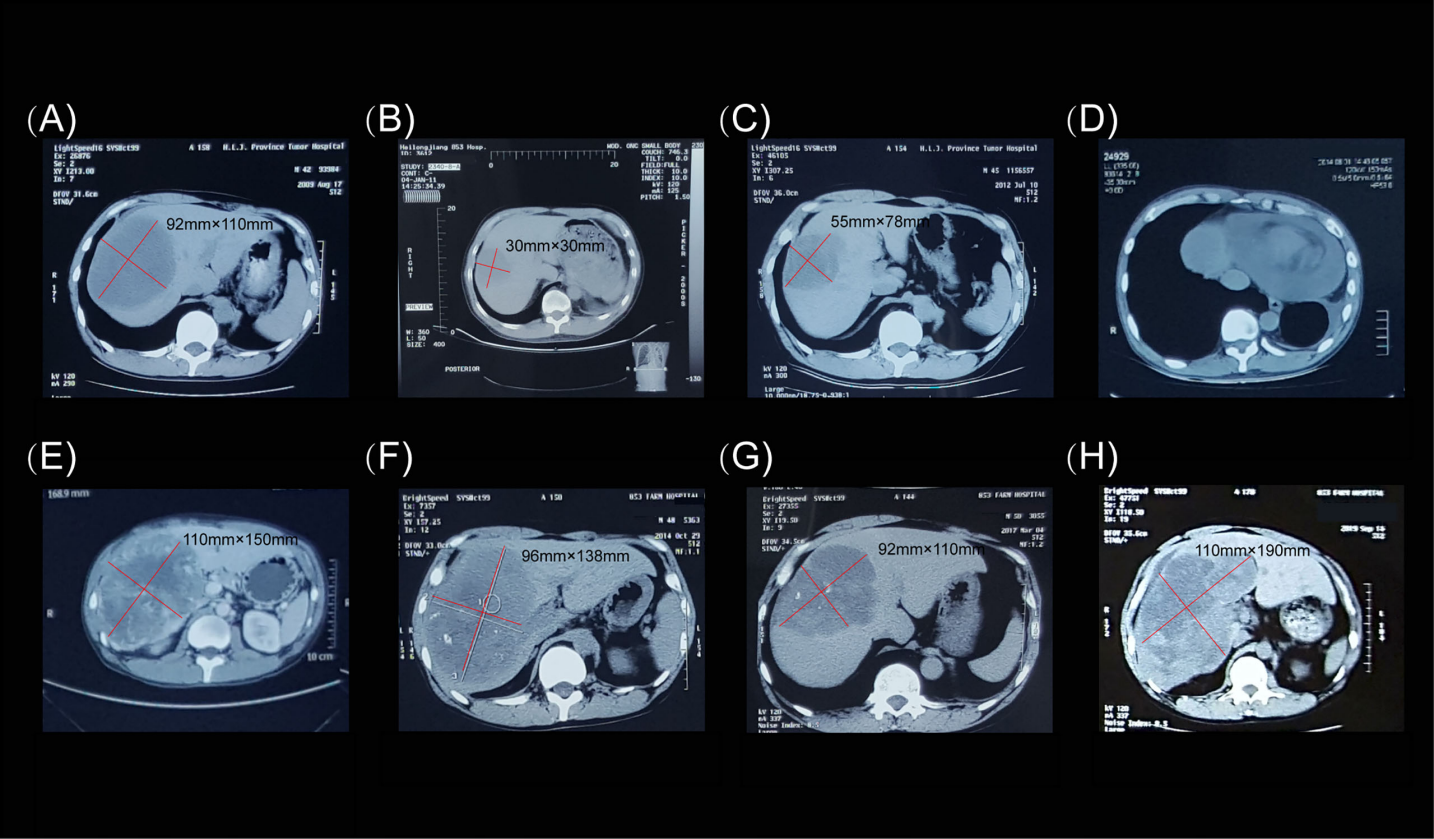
Computed tomography images of the hepatic extra-gastrointestinal stromal tumor. (A) In August 2009, prior to imatinib mesylate administration (400 mg once daily), the tumor mass measured 92 × 110 mm. (B) In January 2011, routine computed tomography examination showed that the lesion in the right hepatic lobe had shrunk to 30 × 30 mm. (C) In July 2012, the lesion grew to 55 × 78 mm. (D) Pericardial effusion was observed in August 2014. (E) In September 2014, the lesion measured 110 × 150 mm. (F) Before the patient was switched to sunitinib malate therapy (50 mg once daily on a 4/2 schedule), the tumor mass measured 96 × 138 mm. (G) In March 2017, the tumor was 92 × 110 mm in size. (H) The final computed tomography examination in October 2019 showed that the tumor mass measured 110 × 190 mm.
